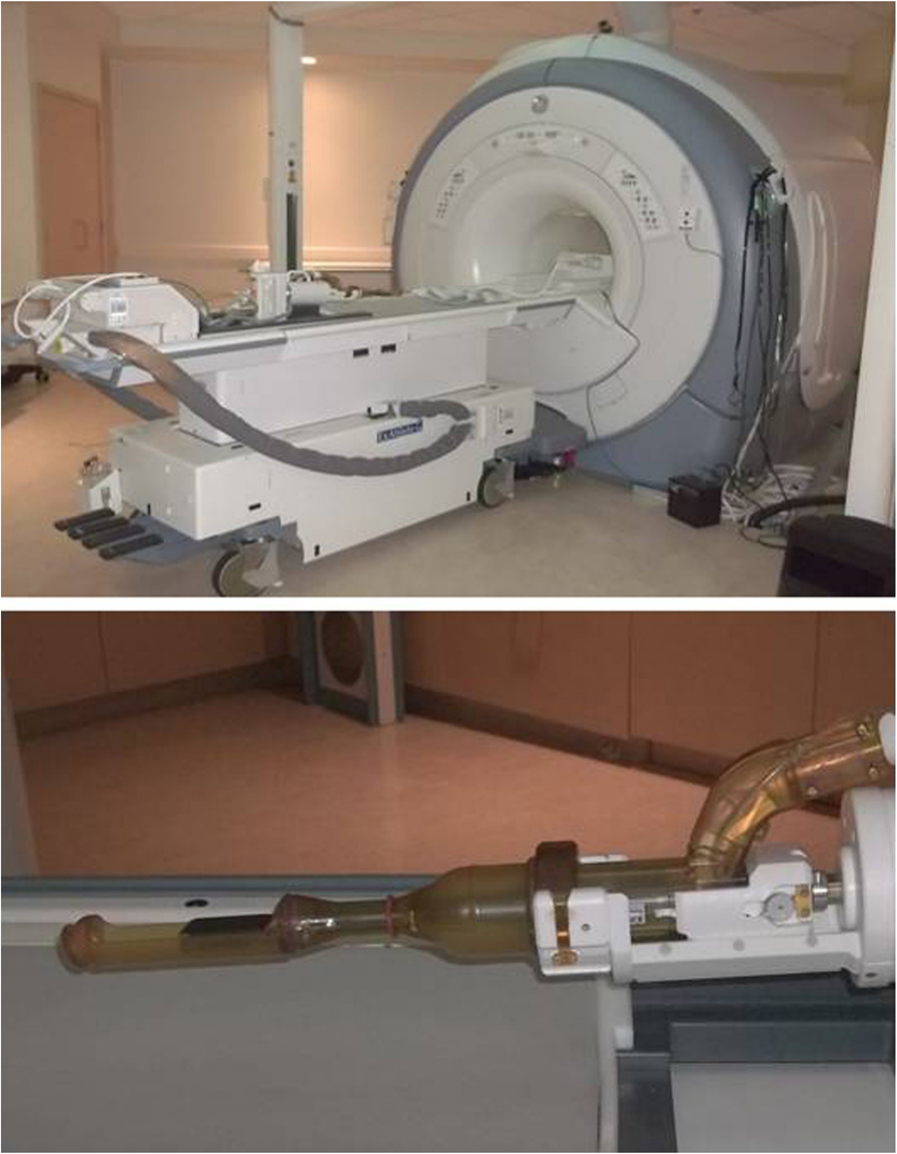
ExAblate 2100 treatment table docked to the MRI scanner (*top*). Trans-rectal ultrasound transducer used to deliver therapy (*bottom*) which consists of a 990-element phased array transducer covered by an endorectal balloon containing circulating chilled (14 °C degassed water)
